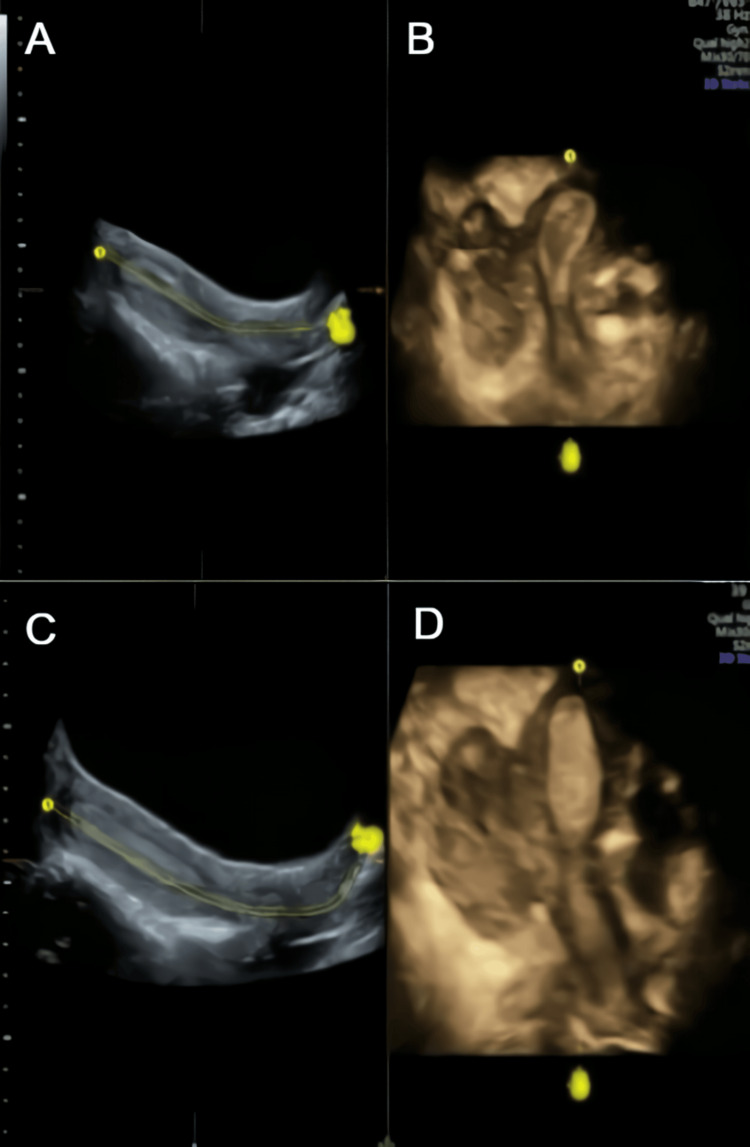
Three-dimensional ultrasonography findings. (A-C) Sagittal view showing a unicornuate uterus with a non-communicating rudimentary horn that was not separated from the uterine surface. (D) Sagittal view showing two separate endometrial cavities that indicated a unicornuate uterus with a non-communicating rudimentary horn.
